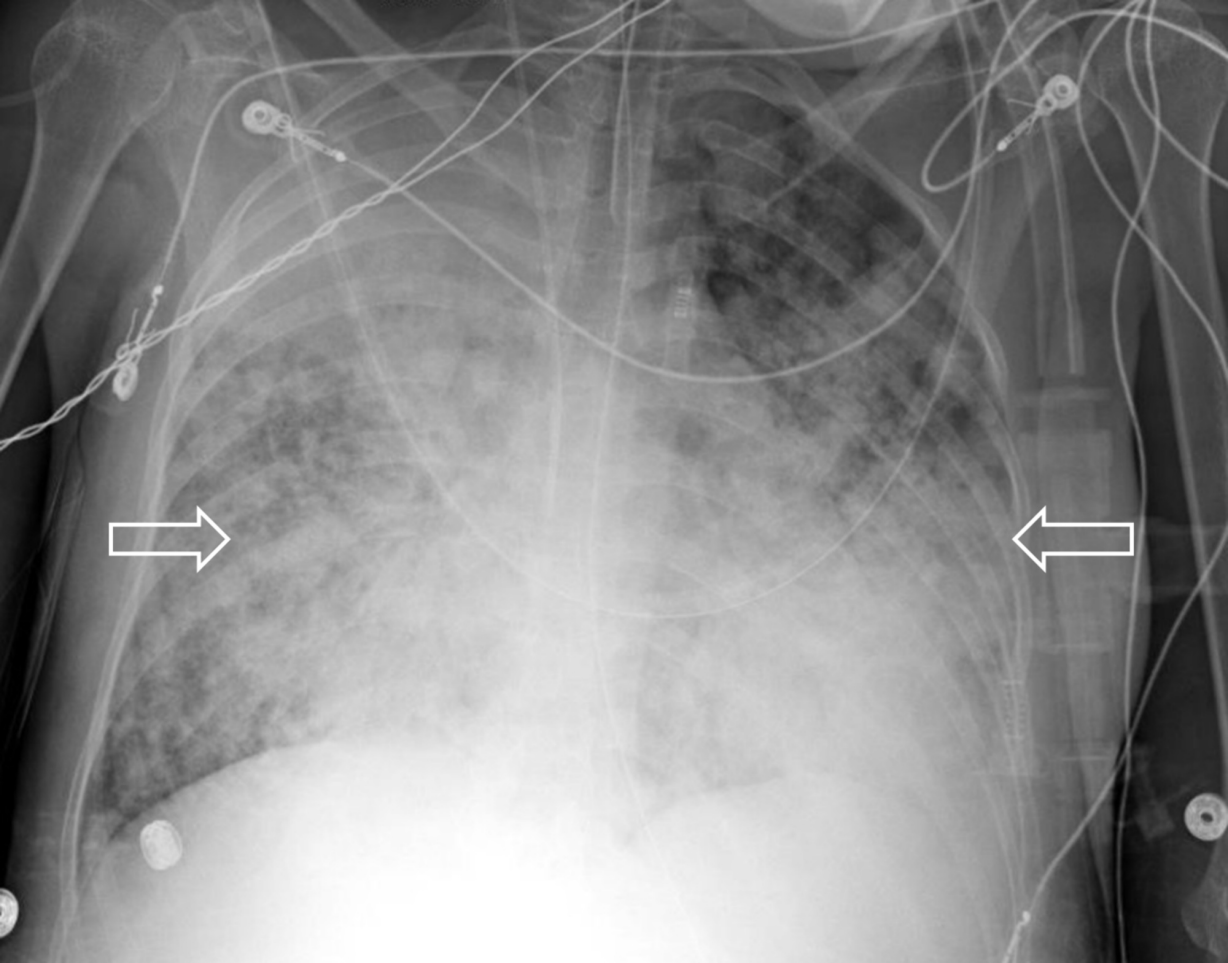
Chest X-ray on hospital day seven, revealing worsening ARDS and DAH (white arrows). ARDS, acute respiratory distress syndrome; DAH, diffuse alveolar hemorrhage
